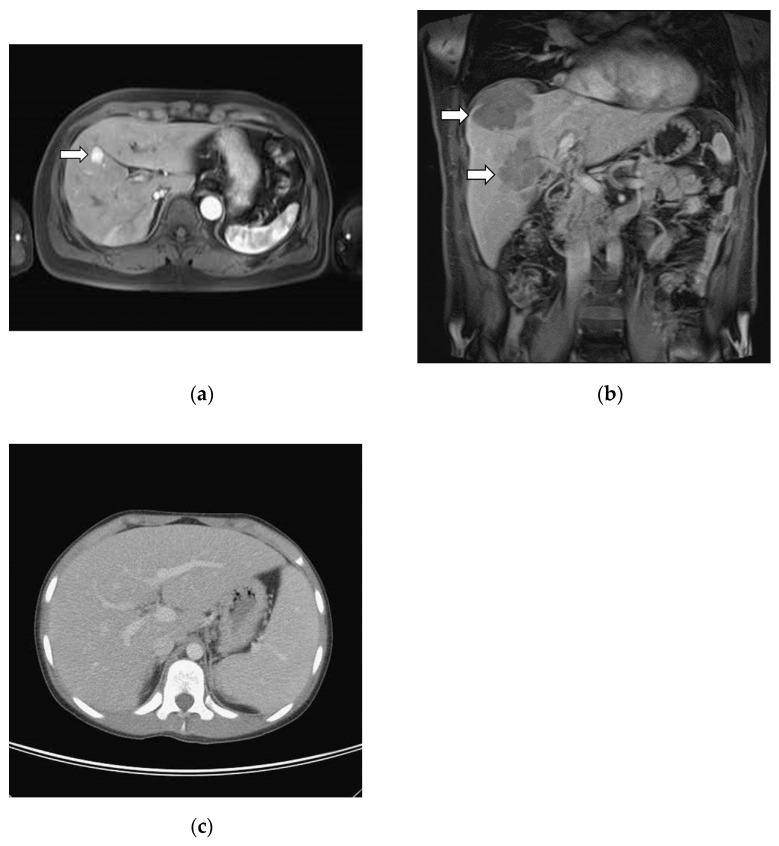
Variable radiographic findings in primary hepatic lymphoma: (a) Abdominal magnetic resonance imaging (MRI) axial T1-weighted fat-saturated image with contrast enhancement, arterial phase. MAGNETOM SymphonyTim 1.5T MRI scanner (Siemens, Erlangen, Germany). This case underwent segmental resection and final diagnosis is primary liver low grade follicular lymphoma (Figure 2a,b). Patient is still alive without evidence of disease 7 years after initial diagnosis; (b) Abdominal MRI coronal T1-weighted fat-saturated image with contrast enhancement, venous phase, from 55-year-old male. MAGNETOM Aera 1.5T MRI scanner (Siemens, Erlangen, Germany). Multiple liver mass lesions (arrow) were found but no other lymphadenopathy. Biopsy of liver showed diffuse large B cell lymphoma, germinal center B phenotype. Patient was treated with R-CHOP in partial response and was alive 3 years after initial diagnosis; (c) Abdominal computed tomography axial image with contrast enhancement, venous phase. Brilliance 64 CT scanner (Philips, Cleveland, OH, USA). Apparent hepatomegaly and splenomegaly noted from 12-year-old body. Random liver biopsy had sinusoidal atypical T lymphocytes infiltration and clonality studies for TCR-gamma was clonal. Final diagnosis is hepatosplenic T cell lymphoma. Patient is alive without disease 8 years after initial diagnosis.
